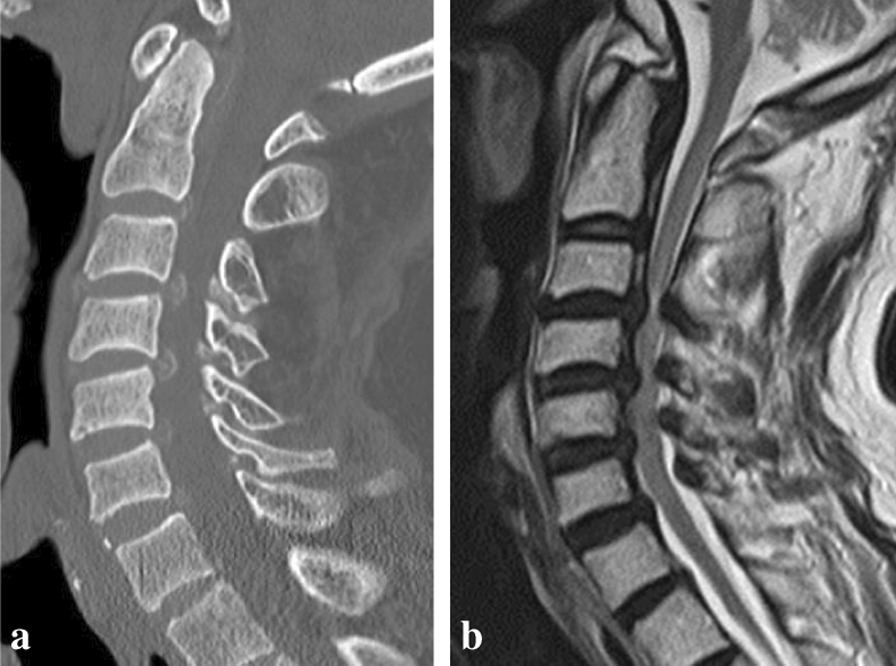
a Computed tomography (CT) showing an extradural amyloid deposit and hypertrophied ligament, which are characteristic findings for dialysis-related spondyloarthropathy. b Magnetic resonance imaging (MRI) showing multilevel spinal cord compression at the C3–C6 levels and T2-weighted hyperintensity of the spinal cord at the C3–C4 level
